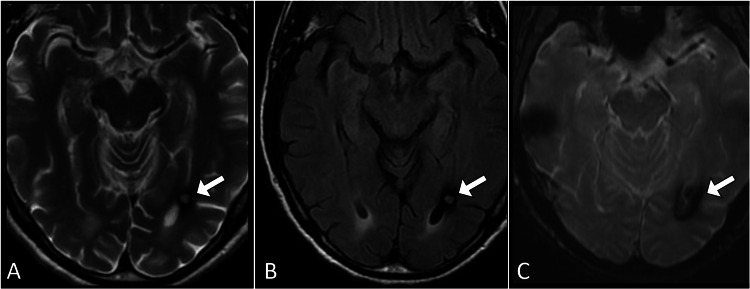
Axial MRI brain images including T2-weighted (A), FLAIR (B), and gradient echo (C) revealing a subependymal lesion (arrow) at the occipital horn of the left lateral ventricle. The lesion exhibits high signal intensity on T2 and FLAIR (A and B) with a surrounding rim of low signal intensity. Additionally, the gradient echo image (C) displays a blooming artifact, characteristic of cavernoma. FLAIR: fluid-attenuated inversion recovery.
